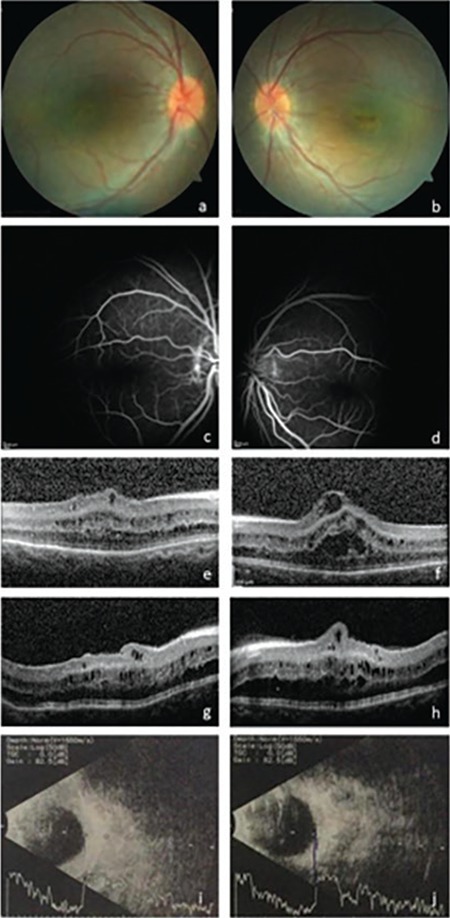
Color fundus photo: mild retinal pigmentary changes and horizontal papillomacular folds bilaterally (a, b). Fundus flourescein angiography: no vascular pathology or cystoid macular edema in both eyes (c, d). Spectral domain optical coherence tomography: retinal folds affecting the neural retina in particular, without involvement of the RPE-choroid band and macular cystoid lesions resembling schisis bilaterally before treatment (e, f) and reduction in cystoid lesion area after treatment (g, h) B-scan ultrasound: diffuse thickening of the choroid and sclera with foreshortening of the globe in both eyes (i, j)
